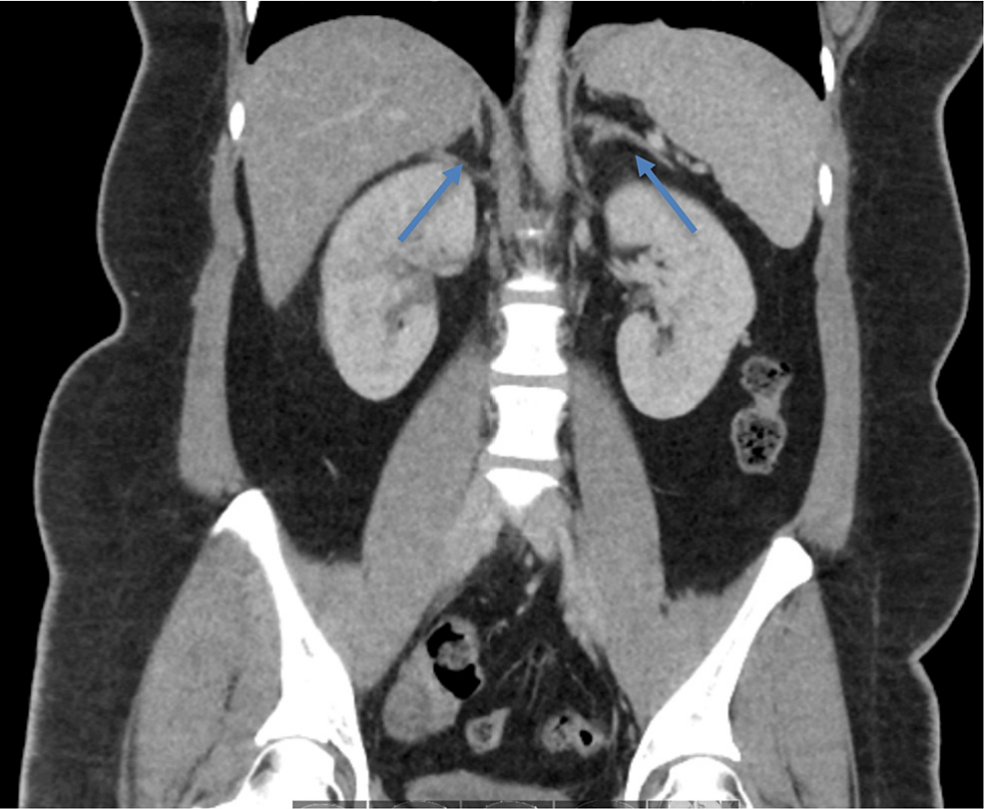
CT of the abdomen negative for any pathology or mass Arrows point to adrenal glands bilaterally. CT: computed tomography
